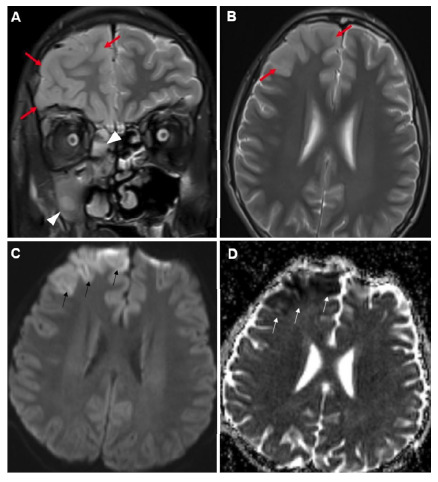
Coronal (A) and axial (B) section T2-weighted brain MRI scans show greater intensity in the right maxillary sinus and ethmoid cells consistent with sinusitis (arrowheads) and diffuse thickness and greater intensity in the gray matter in the right frontal lobe (red arrows). Axial diffusion-weighted images show greater intensity in the B-1000 series (C) and hypointense appearance (arrows) in apparent diffusion coefficient mapping (D) in the frontal lobe.
